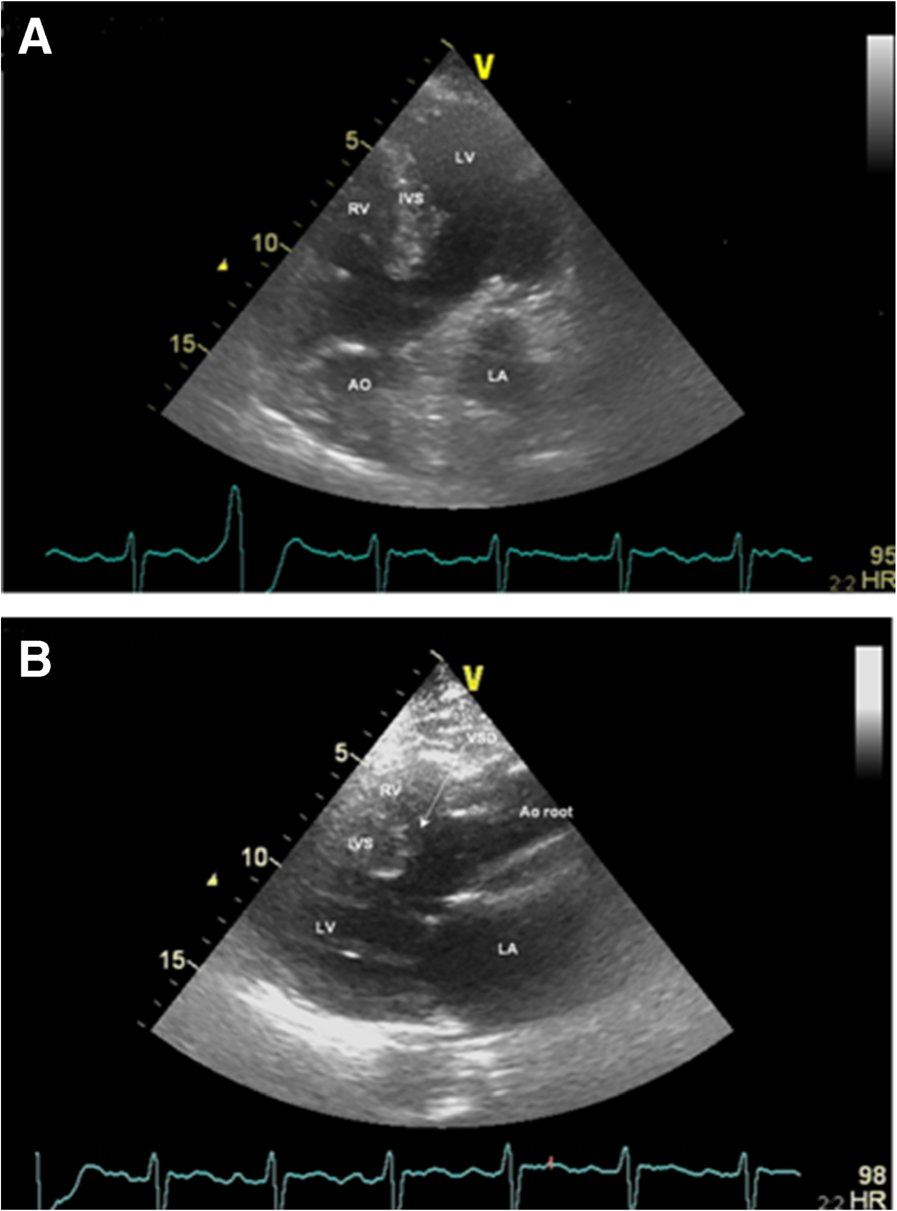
Transthoracic echocardiogram revealing some of the characteristics of tetralogy of Fallot. A: Five chamber view with the presence of the ventricular septal defect (VSD) and the overriding aorta over the septum. B: Parasternal long axis view revealing the VSD, the overriding of the aorta over the septum and also the right ventricle (RV) hypertrophy. AO, aorta; IVS, interventricular septum; LA, left atrium; LV, left ventricle.
